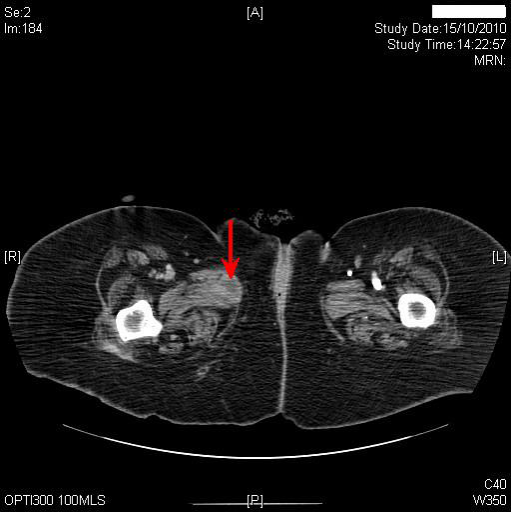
Computed tomography of abdomen, performed on the same day as Figure 2, revealed a collection measuring 2.6 cm × 2.2 cm at the site of insertion of the right adductor muscle (red arrow).
